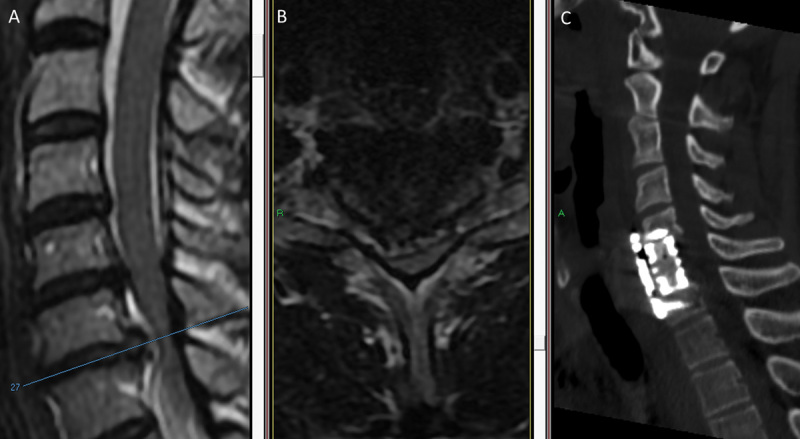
(A) Sagittal and (B) axial T2-weighted magnetic resonance imaging (MRI) examinations demonstrate acute C6–C7 disk herniation with a hyperintense signal in the spinal cord adjacent to the herniated disk. Cord compression is more severe on the right. (C) Postoperative computed tomography (CT) demonstrates implantation of a titanium mesh cage replacing the C6 body and dynamic plate.
